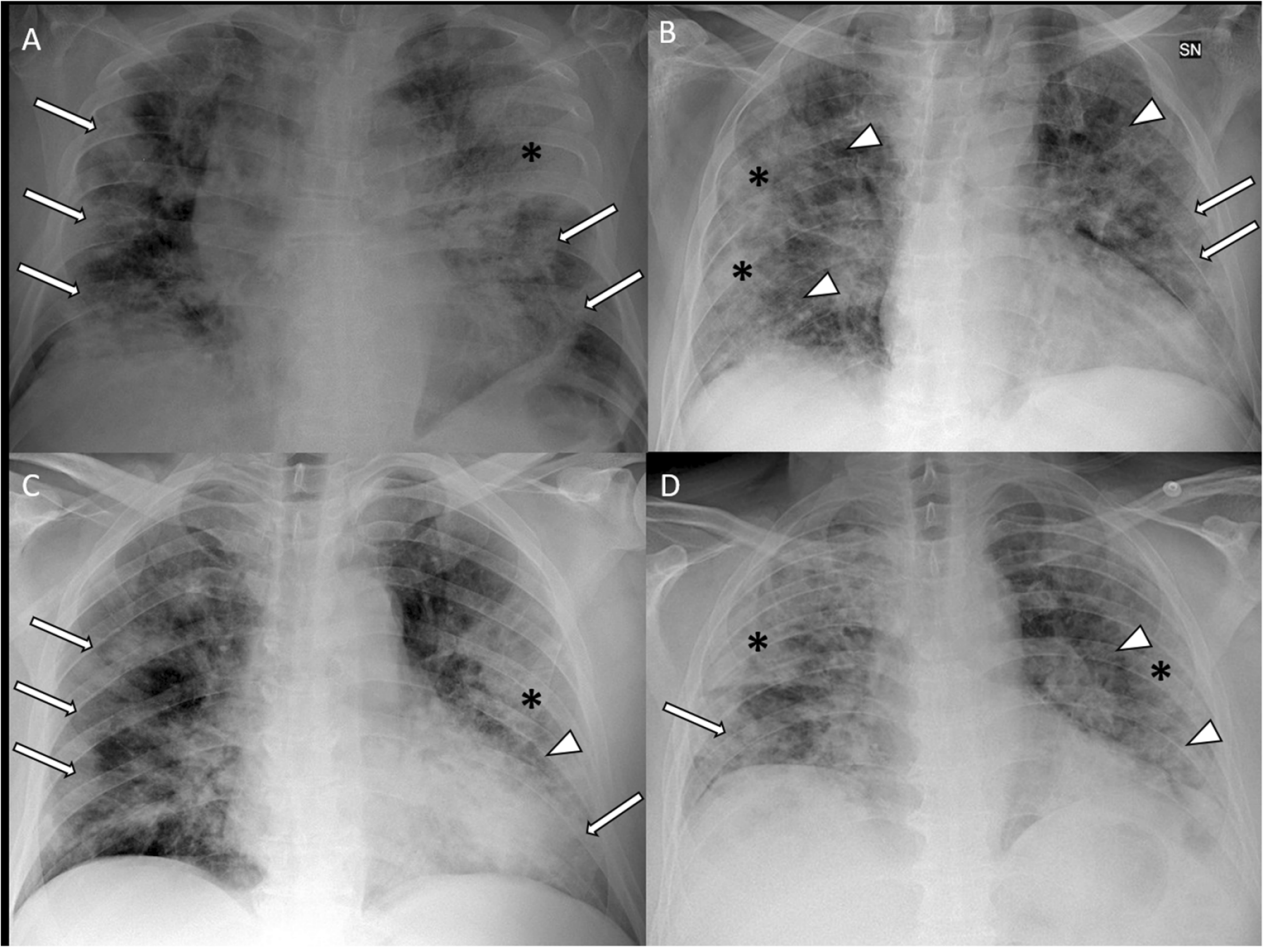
Chest radiography of four different confirmed COVID-19 patients, obtained at admission to the emergency department. CXRs in AP projection (A–D) show bilateral lung involvement with typical radiological patterns of COVID-19 pneumonia including the presence of ground-glass opacities (arrow), interstitial reticular alterations (arrowhead), and extensive consolidations (asterisk). All CXRs were interpreted as positive for COVID-19 by the expert reader and by residents. All four patients had positive rRT-PCR results
